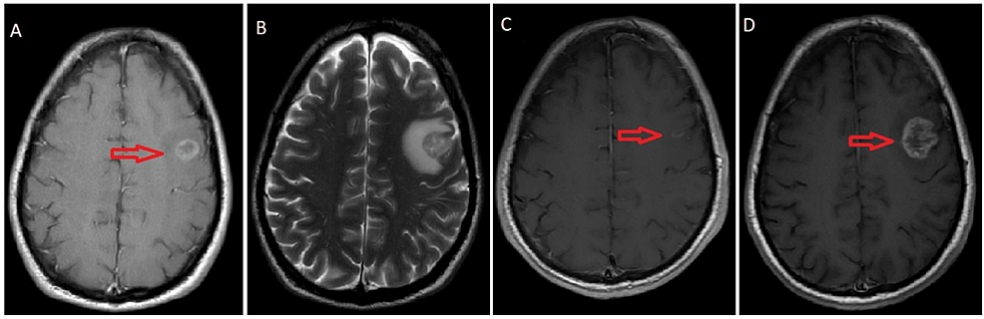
MRI brain images: A and B: pre-treatment brain MRI, T1- and T2-weighted contrast-enhanced; C: follow-up MRI of the brain four months post-FSRT; D: follow-up MRI of the brain one year post-FSRT shows interval progression in the size of the left frontal lesion compared to the post-FSRT response scan. FSRT: fractionated stereotactic radiotherapy
